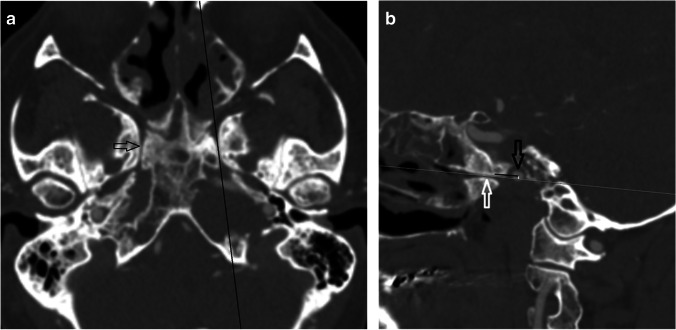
Patient 3. (a) Axial CT angiogram (CTA) on bone windows. Black line on the left demonstrates the sagittal oblique reformat plane for depiction of the relationship of the pICA with the VC. Open white arrow on the right indicates the VC. (b) Sagittal oblique reformat of the CTA on a normal contralateral side. The VC (open white arrow) and the pICA (open black arrow) are demonstrated with the trajectory of the canal indicated by the thin white line. The antero-posterior measurement is demonstrated as the distance from the mid-posterior opening of the VC (in the trajectory of the canal) to the mid AP point of the pICA cross-section (horizontal black line). The craniocaudal measurement is indicated as the vertical perpendicular distance from a line extrapolated posteriorly from the VC (in the trajectory of the canal) to the central point of the pICA cross-section (white vertical line). The line extrapolated from the posterior opening of the VC (in the trajectory of the canal) intersects the pICA (black open arrow)
